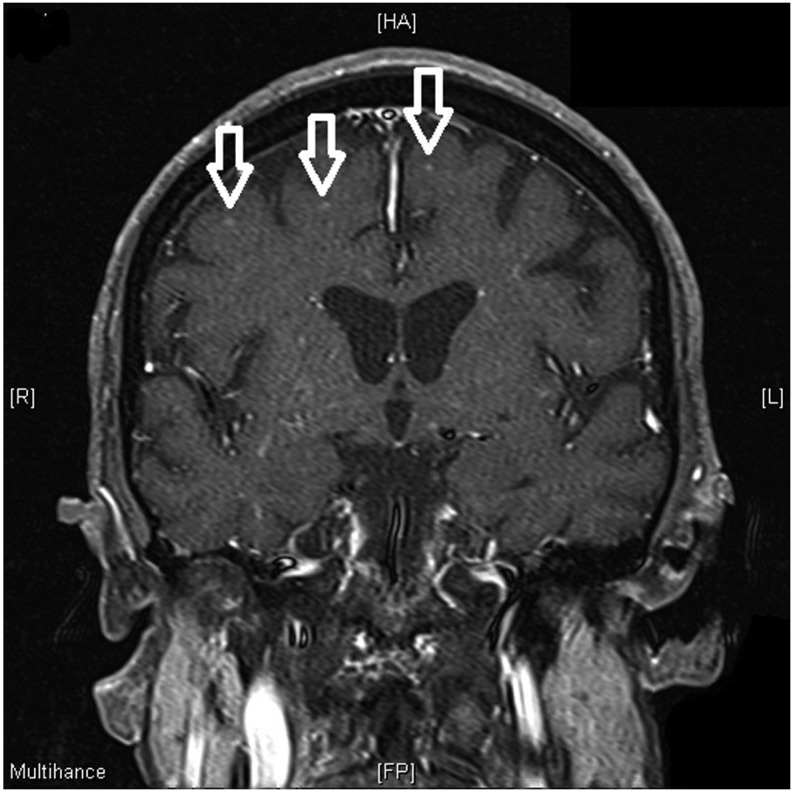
T1-weighted coronal magnetic resonance image of a patient (patient 4) with anti-N-methyl D-aspartate receptor encephalitis and a mRS score of 5 at that time depicting contrast enhancement (white arrows) and subcortical T2-hyper-intensities (T2-weighted image not shown).
